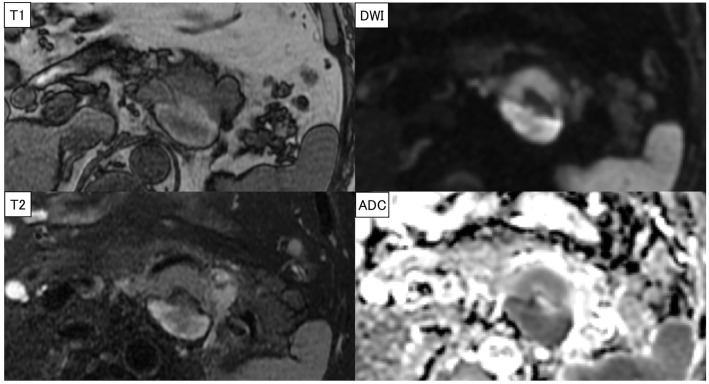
MRI findings. The ventral side of the tumor showed a low signal on T1-weighted images, mildly high signal on T2-weighted images, and high signal on DWIs, suggesting a neuroendocrine or serous cystic tumor. The dorsal part of the tumor suggested a hematoma or necrosis. ADC, apparent diffusion coefficient; DWI, diffusion-weighted image
